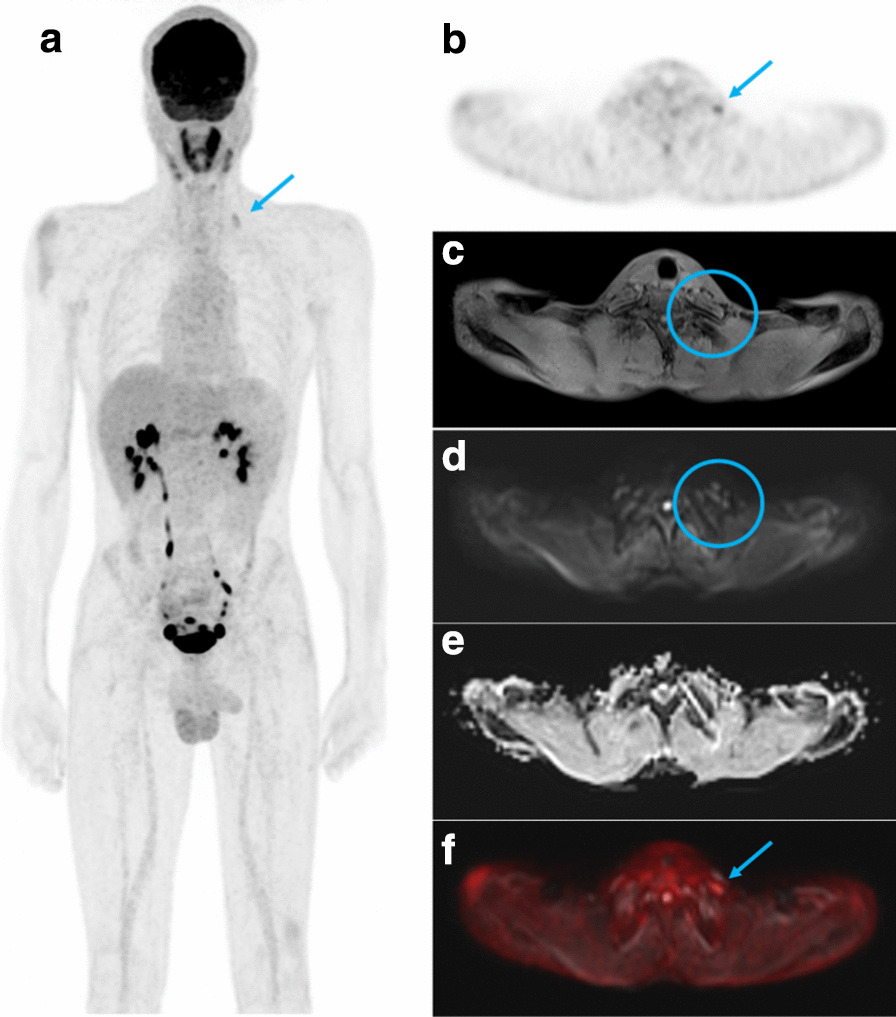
Example of a schwannoma detected by ^[18F]^FDG-PET but not MRI. Left-sided supraclavicular schwannoma (arrows) visible on a coronal maximum intensity projection (MIP) ^[18F]^FDG-PET image and b axial ^[18F]^FDG-PET image. Conversely, the lesion was not detected on c axial T1-weighted fat-suppressed image, d axial b = 800 s/mm^2^ diffusion-weighted (DW) image and e axial apparent diffusion coefficient (ADC) image (circles). f Axial fused ^[18F]^FDG-TEP and b = 800 s/mm2 DW image
